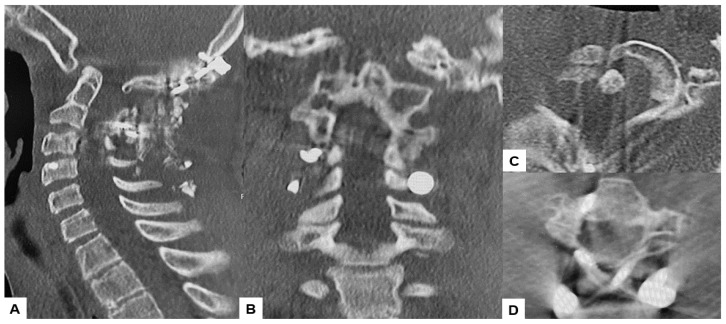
Postoperative CT. (A) Mid-sagittal reconstruction CT, (B) Coronal reconstruction CT, (C) Axial CT at C1/2, (D) Axial CT at C2/3.
